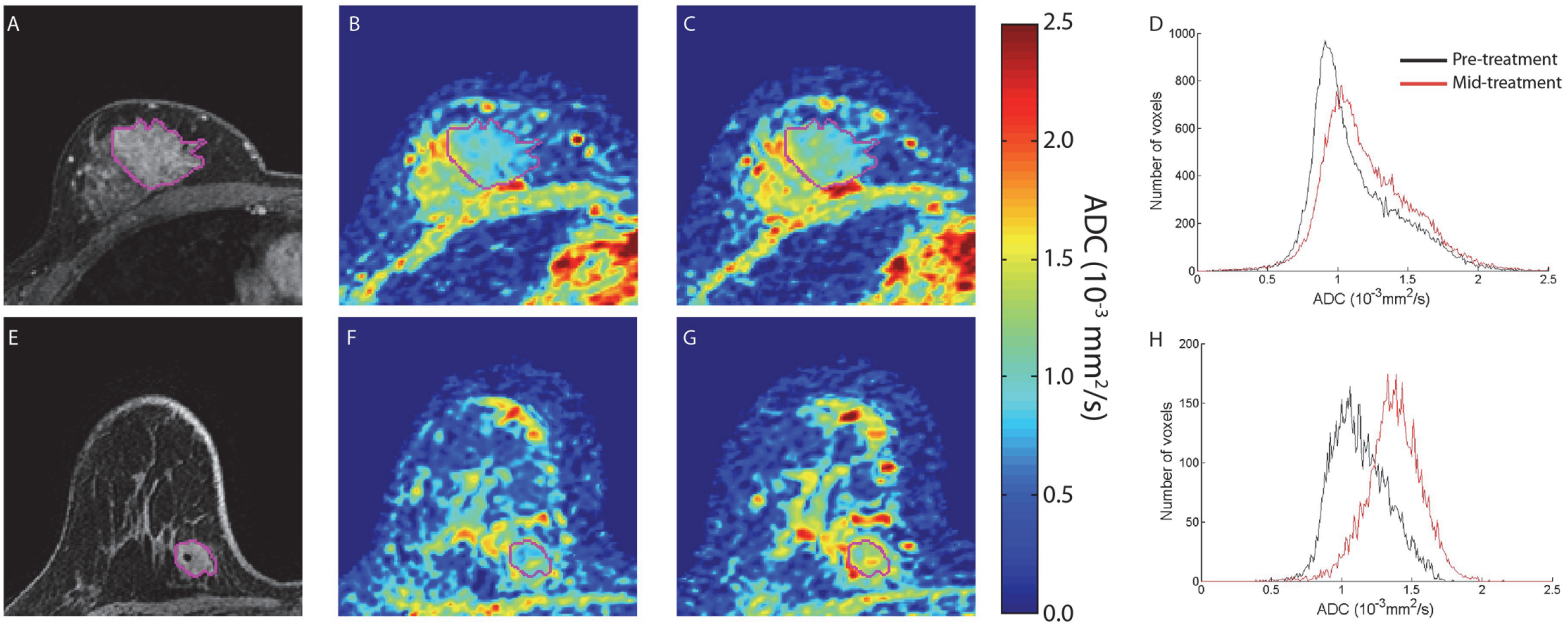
Therapeutic-induced changes in breast tumor ADC values. MRI images are depicted for non-responding (top row) and responding (bottom row) patients treated for breast cancer. (A) and (E) T1-weighted gadolinium enhanced, (B) and (F): pre-treatment ADC maps, (C) and (G): ADC maps at 8–11 days after treatment initiation, D) and (H): Histograms of ADC values in the tumor pre-treatment and post-treatment initiation. Tumor is delineated from surrounding healthy tissue in the individual images by the purple line.
